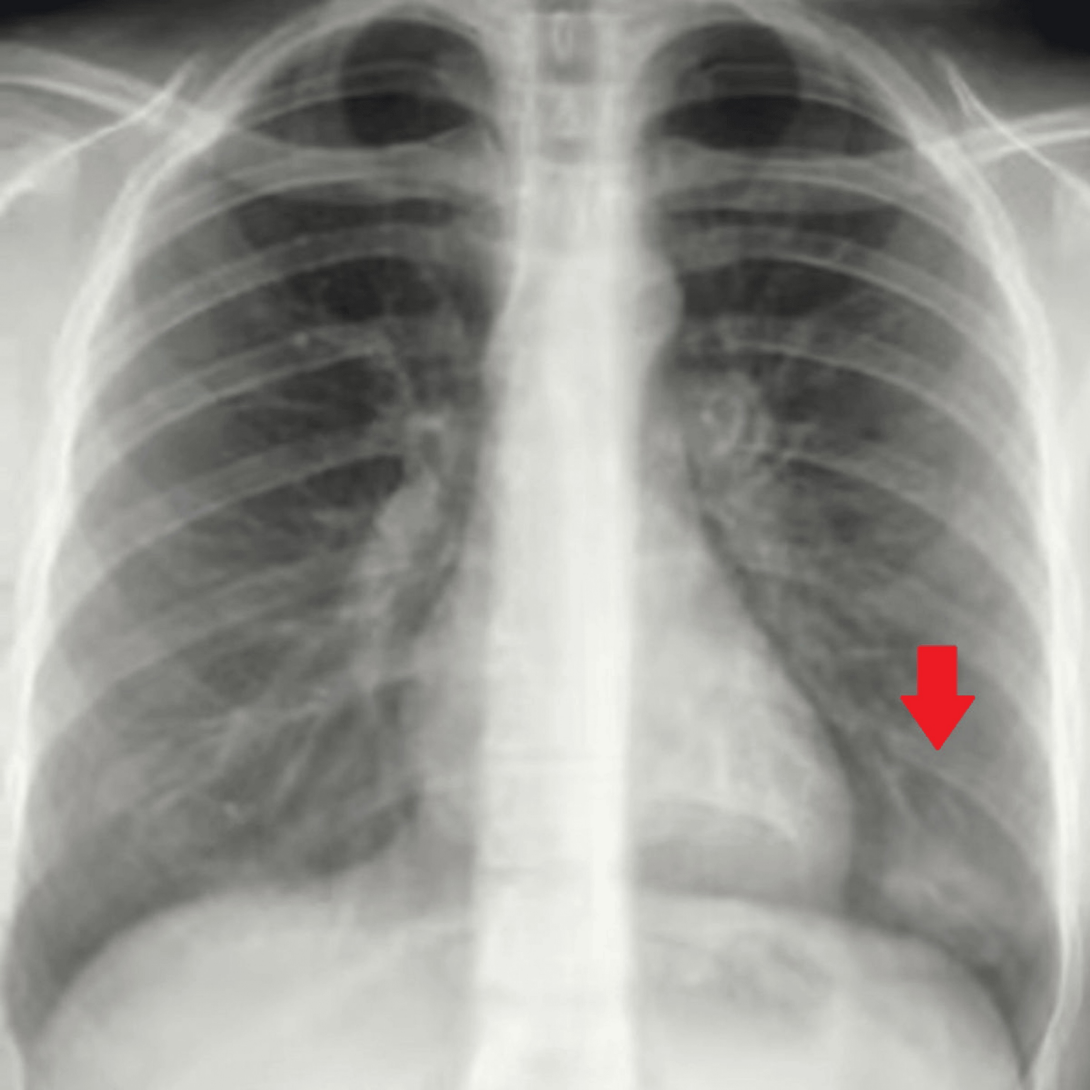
Chest X-ray demonstrating left lower lobe opacity consistent with pneumonia.
